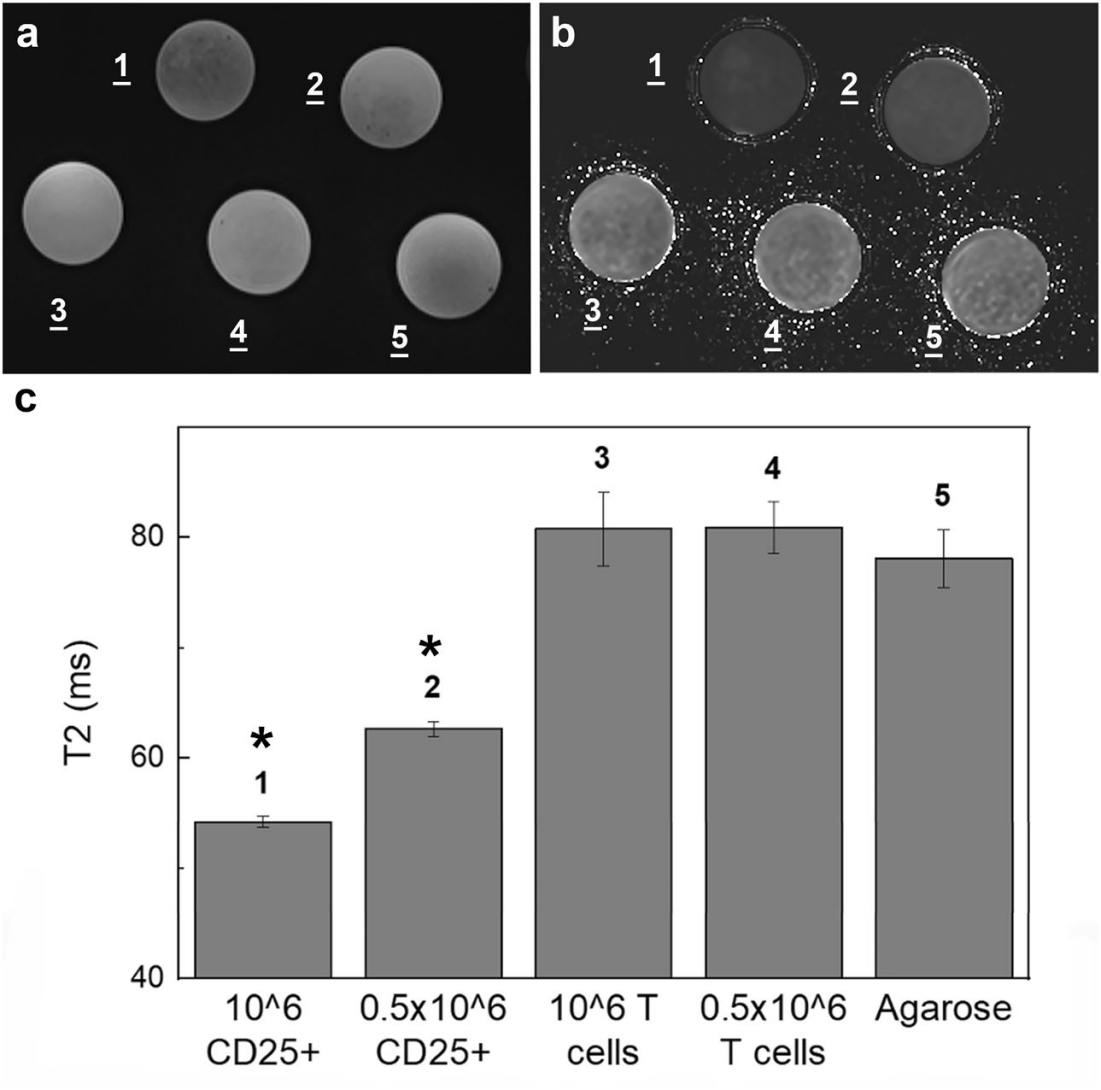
Magnetic resonance signal effects of CD25 microbead-labeled Tregs. T2-weighted magnetic resonance images (a) and corresponding T2 relaxation time maps (b) of agarose gels containing CD25 microbead-labeled Tregs (1–2) show marked signal reduction compared to unlabeled T cells (3–4) and agarose (5) controls. Quantitative analysis of the T2 relaxation times (c) confirms significant T2 shortening in CD25-microbead labeled cells. T2 of half million Tregs is 22% lower than the T2 of unlabeled T cells and agarose alone (P* = 0.0001 and 0.0006 respectively). T2 is further shortened (33%) in one million labeled Treg samples (P* = 0.001 compared to half million Treg sample). Data are presented as mean ± standard deviation.
